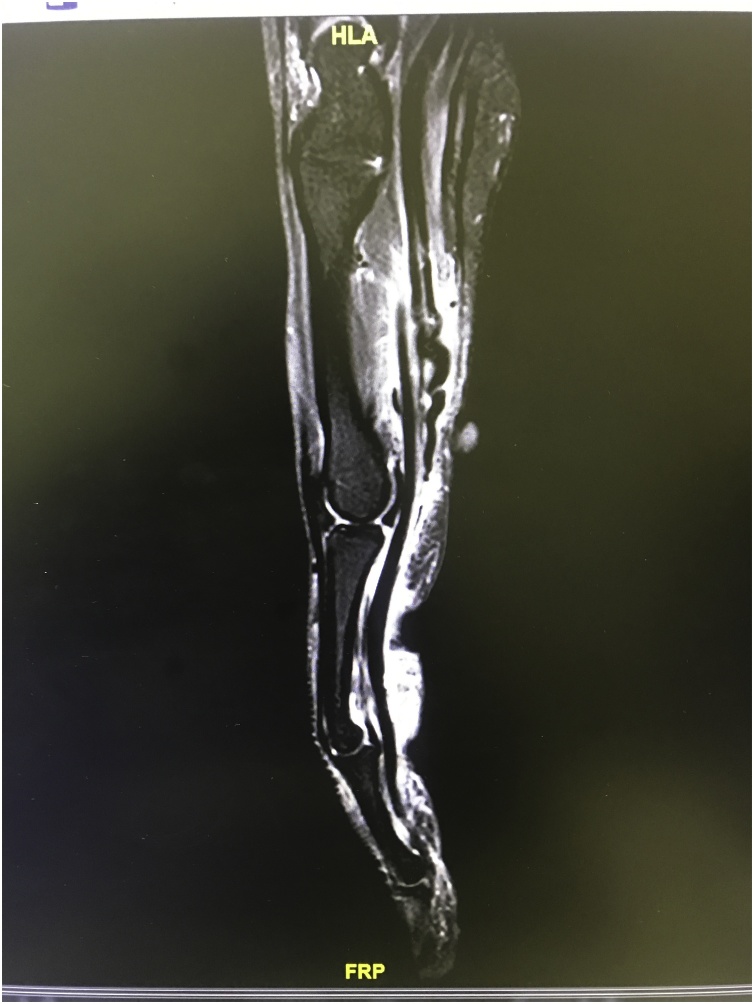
MRI of involved finger showing coiled ruptured FDA tendon.
